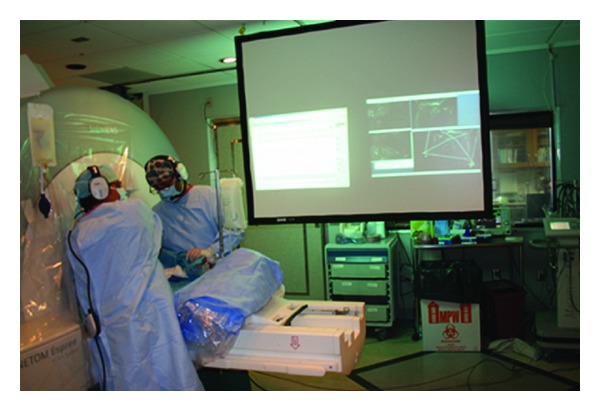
Using real-time MRI as projected onto the screen, the surgeon advances the delivery device into the LV. He can then precisely position the prosthetic valve for deployment.
